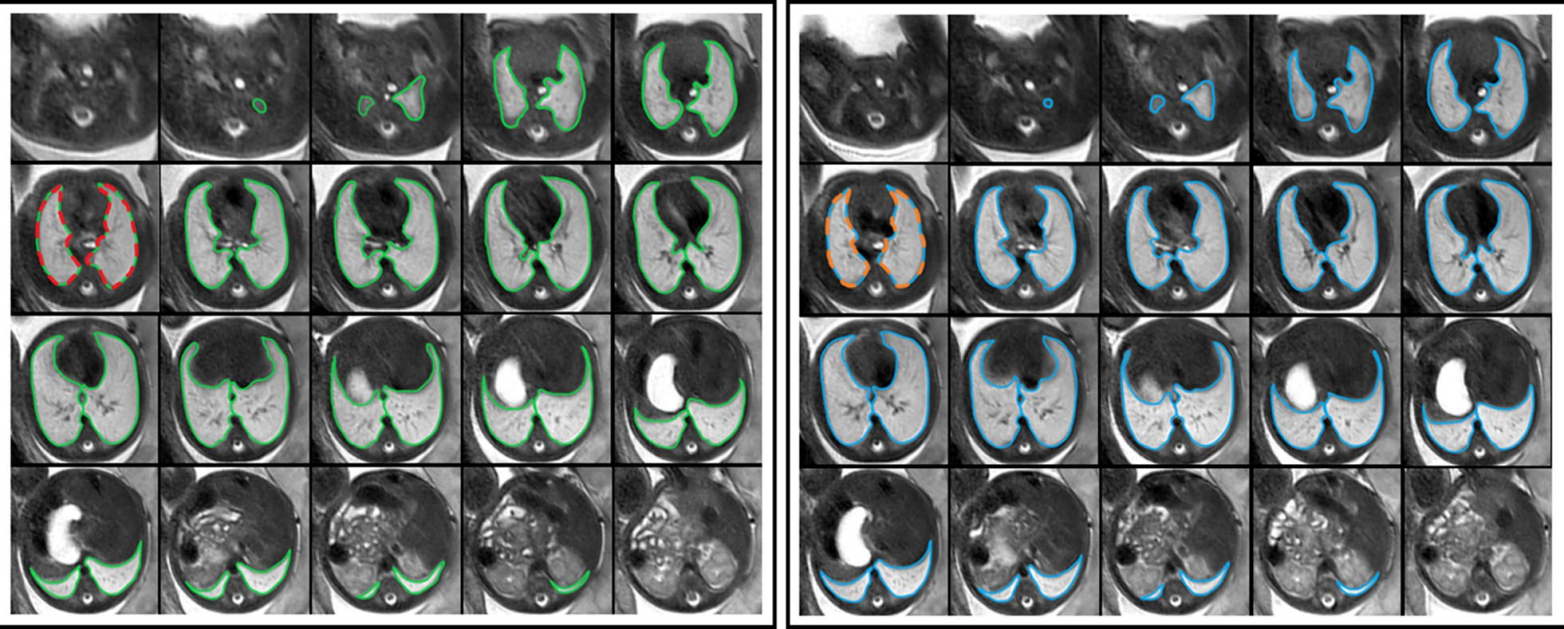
Study design. Repeated acquisitions of T2-weighted axial MRI images of the lung in a fetus at gestational week 32. Three-dimensional (3D) regions of interest (ROIs) encompassing the whole lung were manually segmented in initial (green) and repeat (blue) acquisitions of 30 fetuses. Two-dimensional (2D) ROIs were defined as lung segmentations at the level of the carina in initial (red) and repeat (orange) acquisitions, respectively. Radiomics feature reproducibility was assessed between features extracted from 2 and 3D ROIs in the initial acquisition (red vs. green), 2D ROIs in repeated acquisitions (red vs. orange), and 3D ROIs in repeated acquisitions (green vs. blue)
